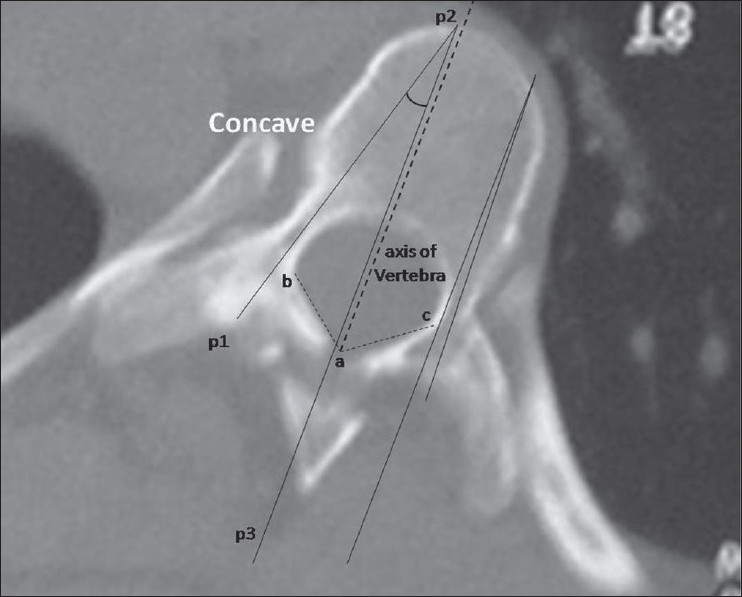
Axial CT image depicting transverse pedicle angle-Concave and convex sides with vertebral axis. It is the angle between the pedicle axis and a line parallel to the vertebral axis in the transverse plane. The vertebral axis is the line bisecting the angle between the lines formed by joining the junction of both laminae posteriorly (a) and one point each on the medial cortical wall where the lamina meets the pedicle (b and c). The transverse pedicle angle is formed by the angle between the pedicle axis p1-p2 and a line parallel to the vertebral axis p2-p3 on the concave side
